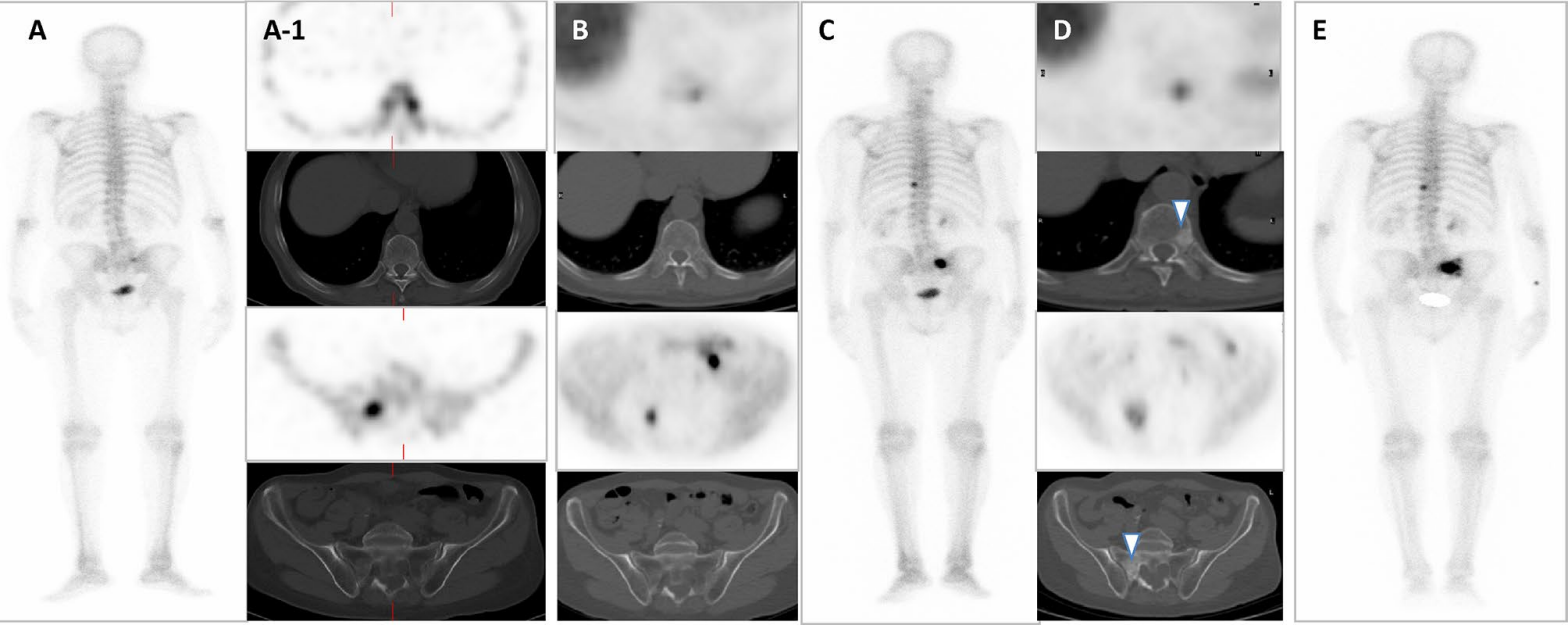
Patient ≠ 1. Baseline total body BS (A) and SPECT/CT (A-1) show oligometastatic disease in dorsal spine and right sacrum. Baseline FCH PET/CT (B) shows the same pathological locations, without alterations in bone density on CT. Interim total body BS (C) showing stability in number of lesions with an increase in osteoblastic activity. Stability regarding the number of lesions on interim FCH PET/CT (D) with an increase of density in CT portion (arrowheads). Clinical progression was diagnosed with clinical impairment plus PSA progression, with an increase in size of known lesions on end-treatment BS (E). 74 years old man, Gleason 9, ^223^Ra in third line, baseline PSA: 32 ng/ml. PFS and OS of 3 and 23 months, respectively.
